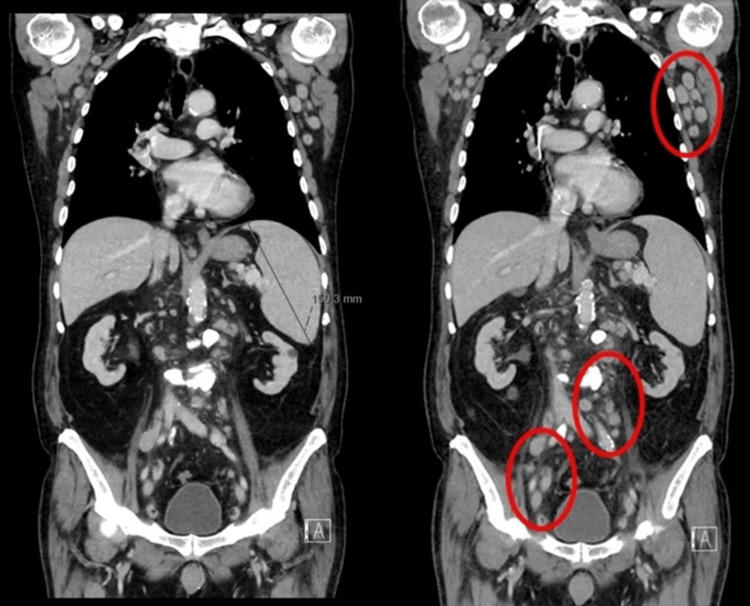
Chest and abdominal computed tomography Adenomegaly (bilateral axillary adenomegaly with slight growth compared to the previous examination, the larger on the left at 25 x 16 mm), small pleural effusion and splenomegaly (15 cm bipolar diameter).
